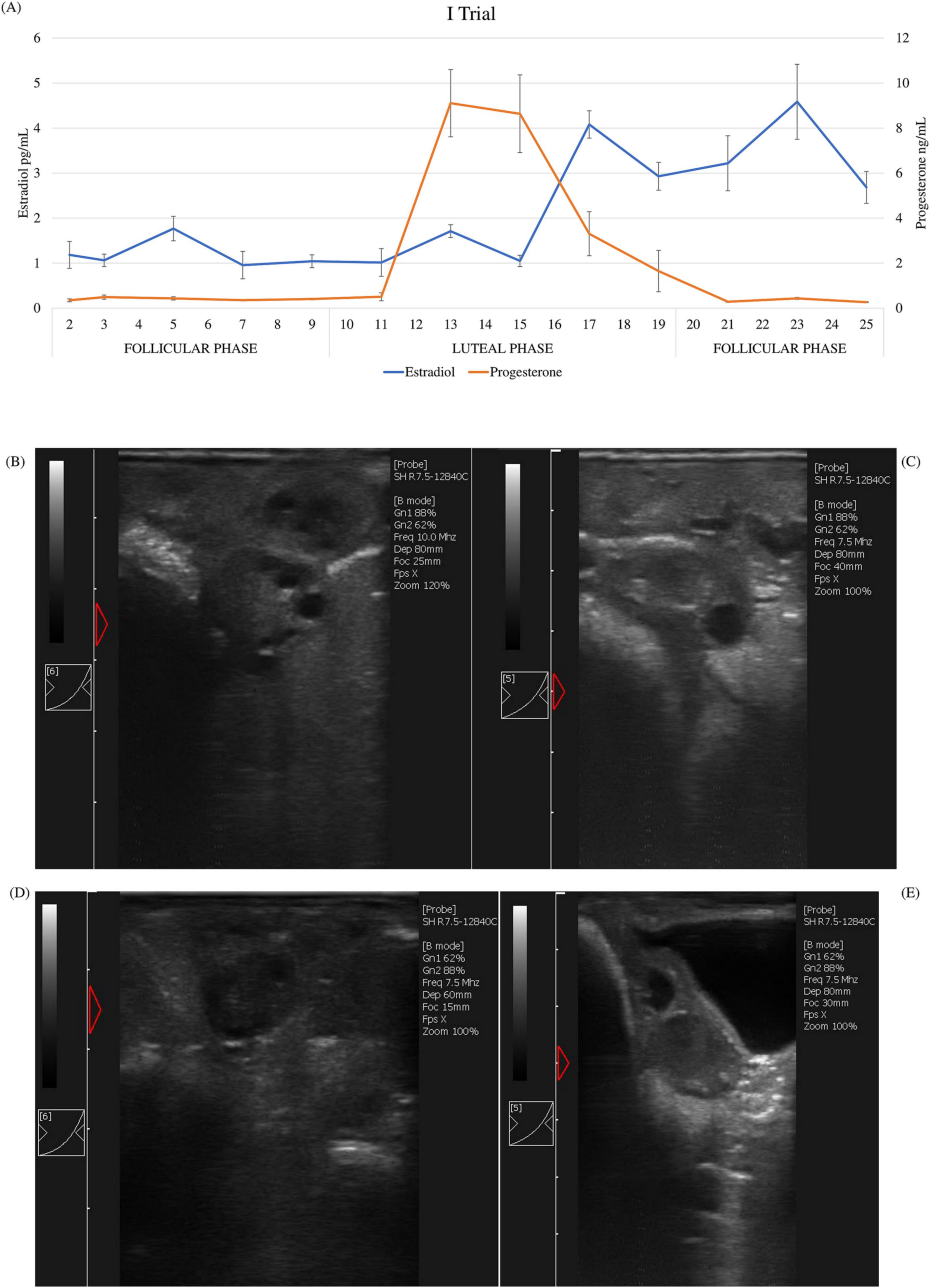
First trial: (A) average of estradiol and progesterone concentrations (±SD) of ewes (*n* = 20) and ultrasound scan images of a representative ewe: (B) growing follicles at day 2; (C) dominant follicle at day 9; (D) early corpus luteum at day 11; (E) corpus luteum at day 15.
